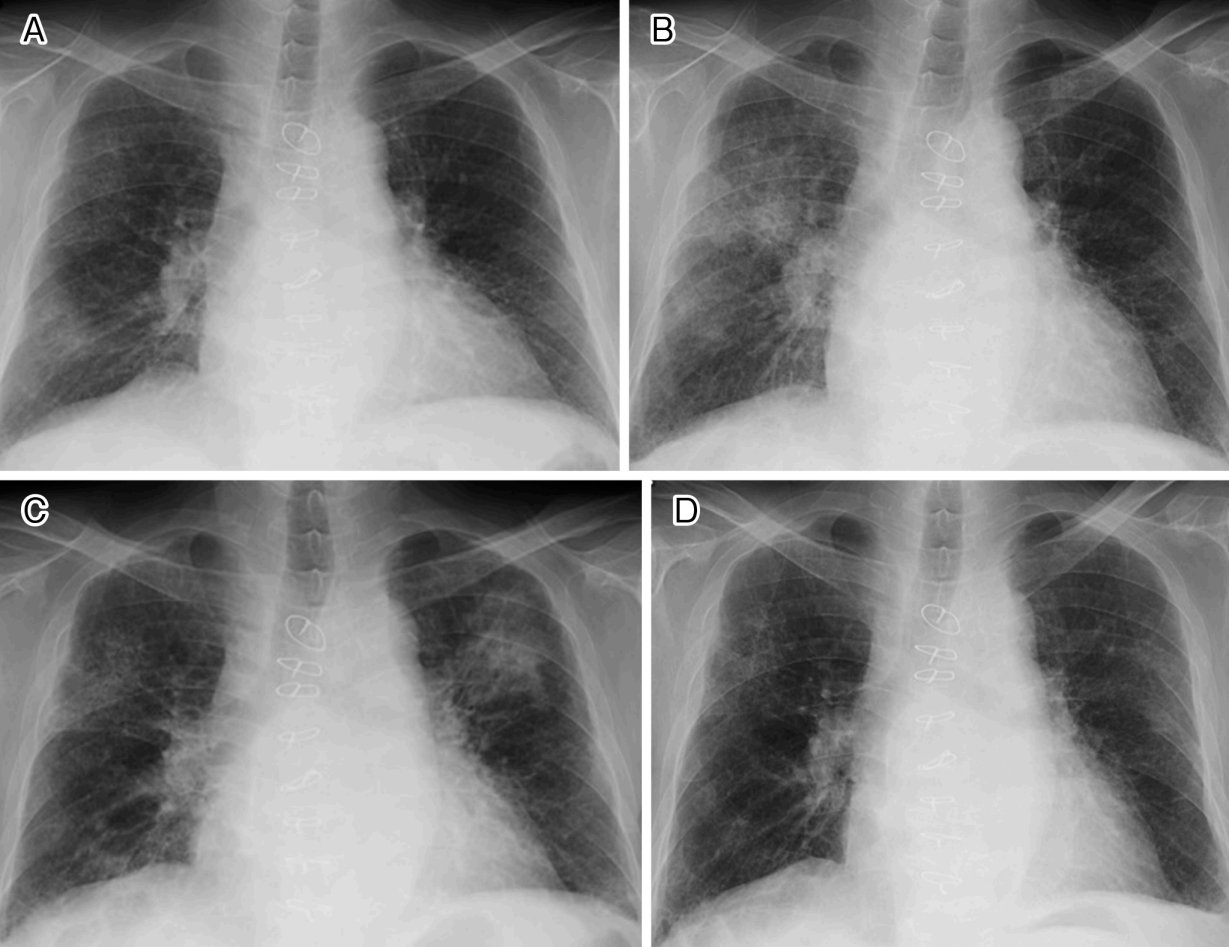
Abnormal shadow in the right lower lung field was observed in the first chest X-ray (1a). The shadow had migrated to the right upper lung on Day 868 (1b). The shadow had migrated in the left upper lung on Day 986 (1c). The shadow had almost disappeared on Day 1,048 (1d).
